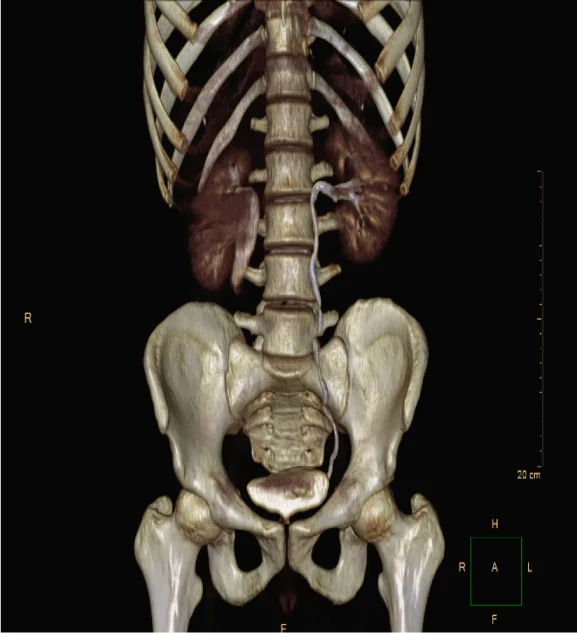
Three‐dimensional reconstructed CT urography demonstrates a right‐sided retrocaval ureter with mild‐to‐moderate proximal hydroureteronephrosis. The proximal ureter shows a posterior and medial deviation consistent with a retrocaval course at the level of the L2 vertebra.
